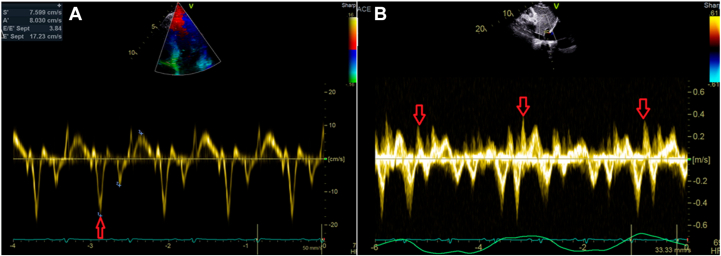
Case 2. Two-dimensional TTE, apical 4-chamber view with tissue Doppler (A) and subcostal view of the hepatic vein with respirometer (B), demonstrates annulus paradoxus with supranormal septal e’ at 17.2 cm/s (*single arrow*) and exaggerated early expiratory diastolic flow reversal in the hepatic vein (*3 arrows*).
